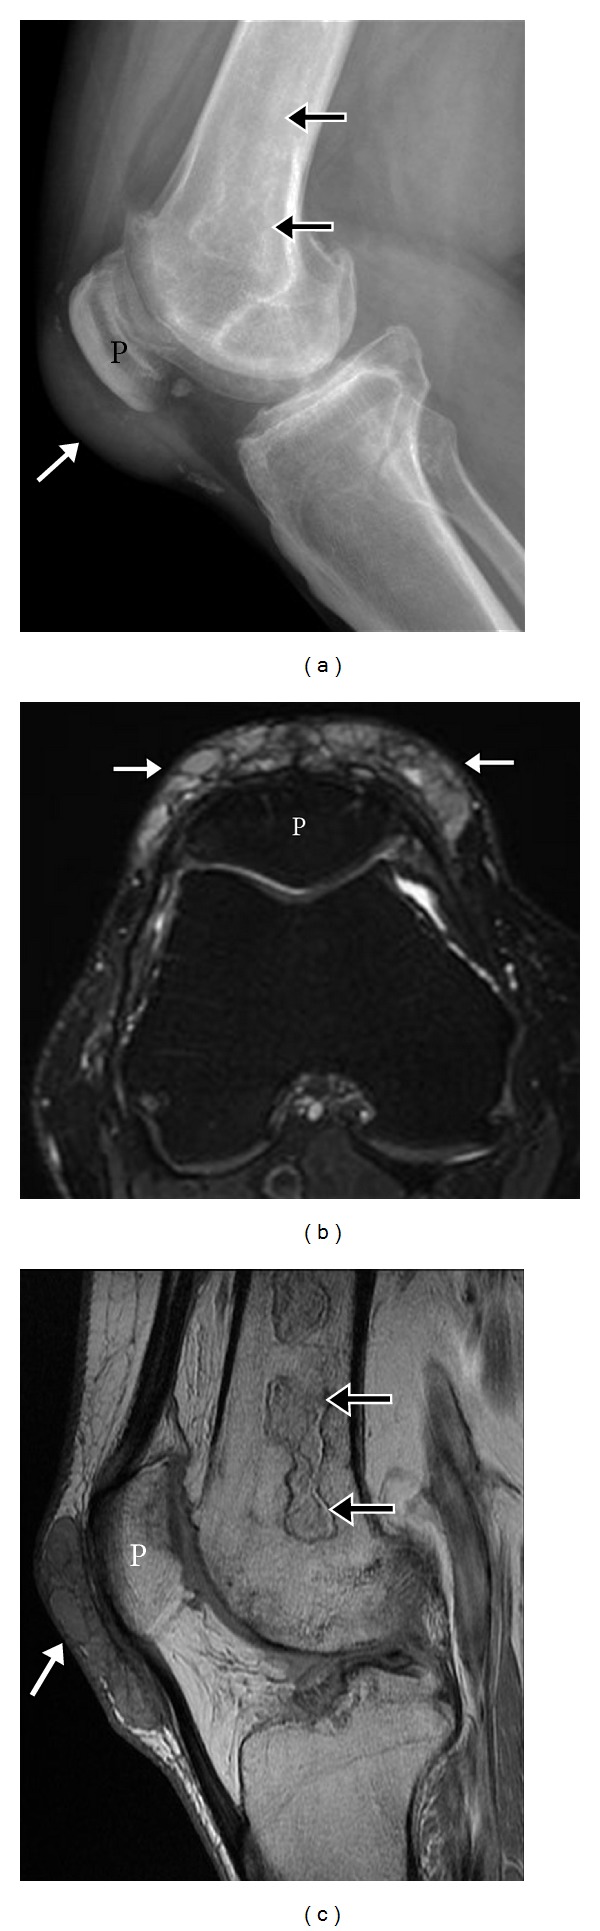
Prepatellar bursal gout. Lateral knee radiograph (a) shows dense focal prepatellar soft tissue swelling with peripheral soft tissue calcifications. Axial T2 (b) and sagittal fat saturated proton density (c) MR images demonstrate intermediate soft tissue corresponding to the prepatellar bursa with internal septations. Incidental note is made of femoral bone infarcts (thin arrow). P: patella.
